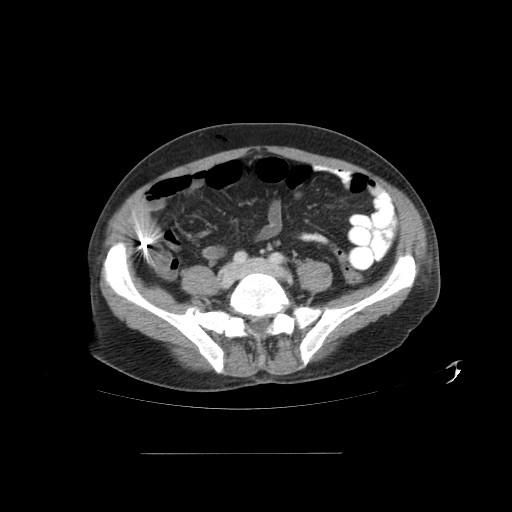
Computed tomography of the abdomen showing a metallic artefact (likely dental amalgam) in appendix region. Scan taken during the initial admission.
